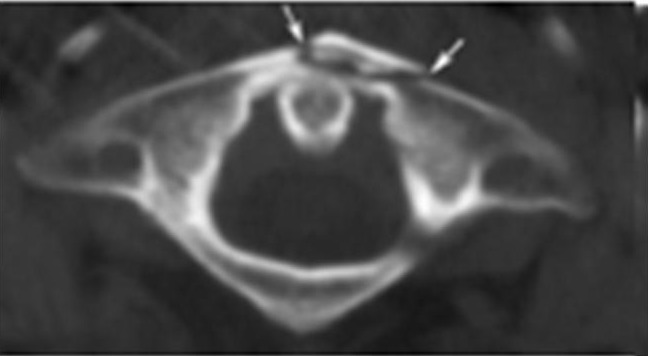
Axial computed tomography demonstrating a traumatic anterior arch atlas fracture
